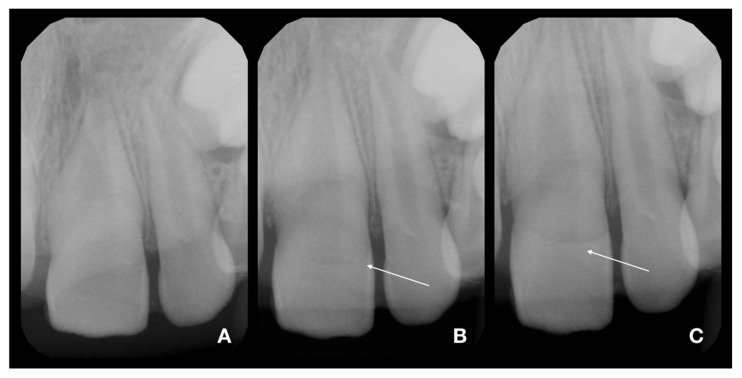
Diagnostic X-rays of teeth 21 and 22 in three different angulations [21]. (A)—foreshortened X-ray (increased angulation of central beam to the direction used in ordinary occlusal exposure). The fracture line is almost not visible. (B)—distortion-free X-ray (angulation used in ordinary occlusal exposures). The fracture line started to be pronounced; see arrow. (C)—elongated X-ray (decreased angulation of central beam to the direction used in ordinary occlusal exposure). The fracture line is clearly visible; see arrow.
